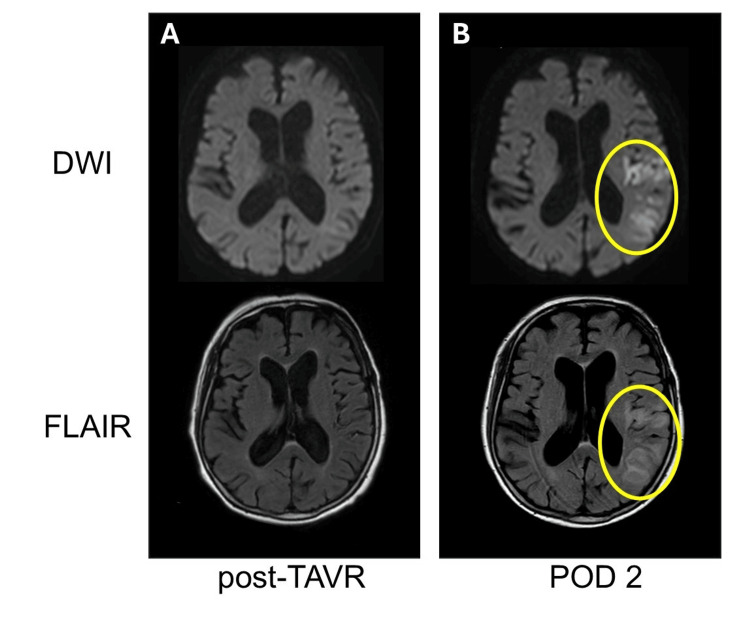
Magnetic resonance images after TAVR and on the second postoperative day DWI: diffusion-weighted image; FLAIR: T2-weighted fluid-attenuated inversion recovery image; POD: postoperative day; TAVR: transcatheter aortic valve replacement. A: The post-TAVR images showed no significant changes in both DWI and FLAIR. B: The POD2 images showed high-signal areas along the ischemic region both DWI and FLAIR.
